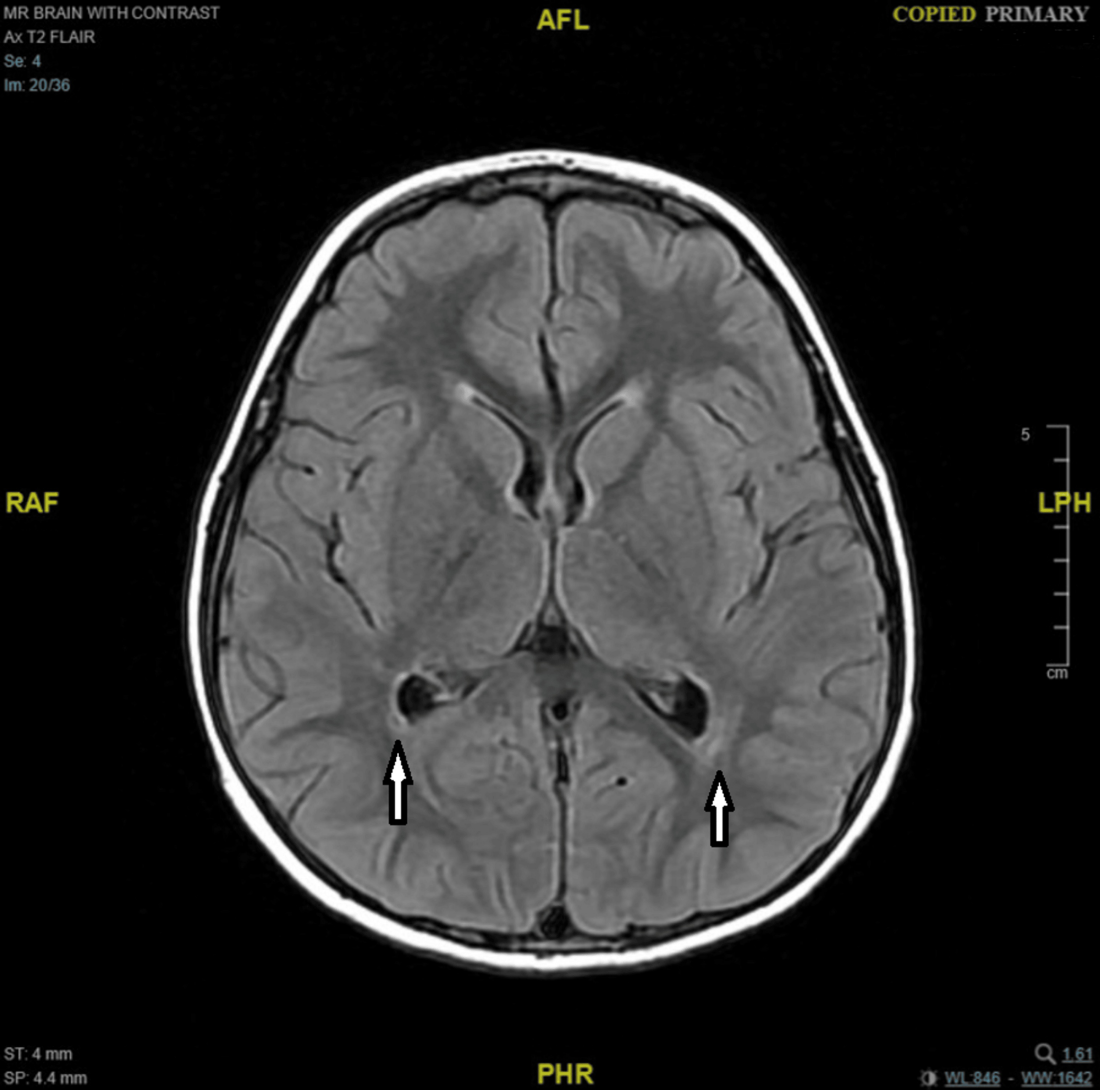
Axial T2/FLAIR brain MRI shows a hyperintense signal involving the peri-trigonal white matter FLAIR: fluid-attenuated inversion recovery; MRI: magnetic resonance imaging; T2: T2-weighted imaging; AFL: anterior-foot-left; RFA: right-foot-anterior; LHP: left-head-posterior; PHR: posterior-head-right
